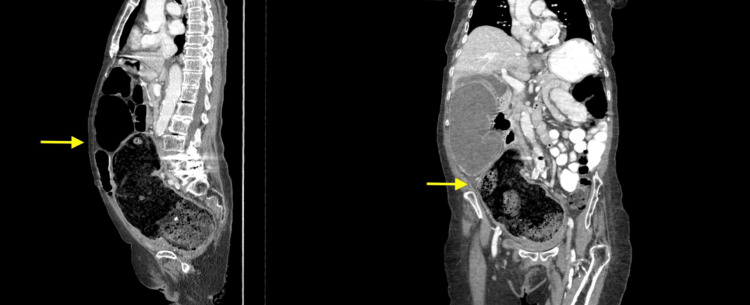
CT abdomen pelvis without contrast was read as marked colonic distension, particularly pronounced in the rectosigmoid region, which is consistent with constipation and fecal impaction (yellow arrow).
